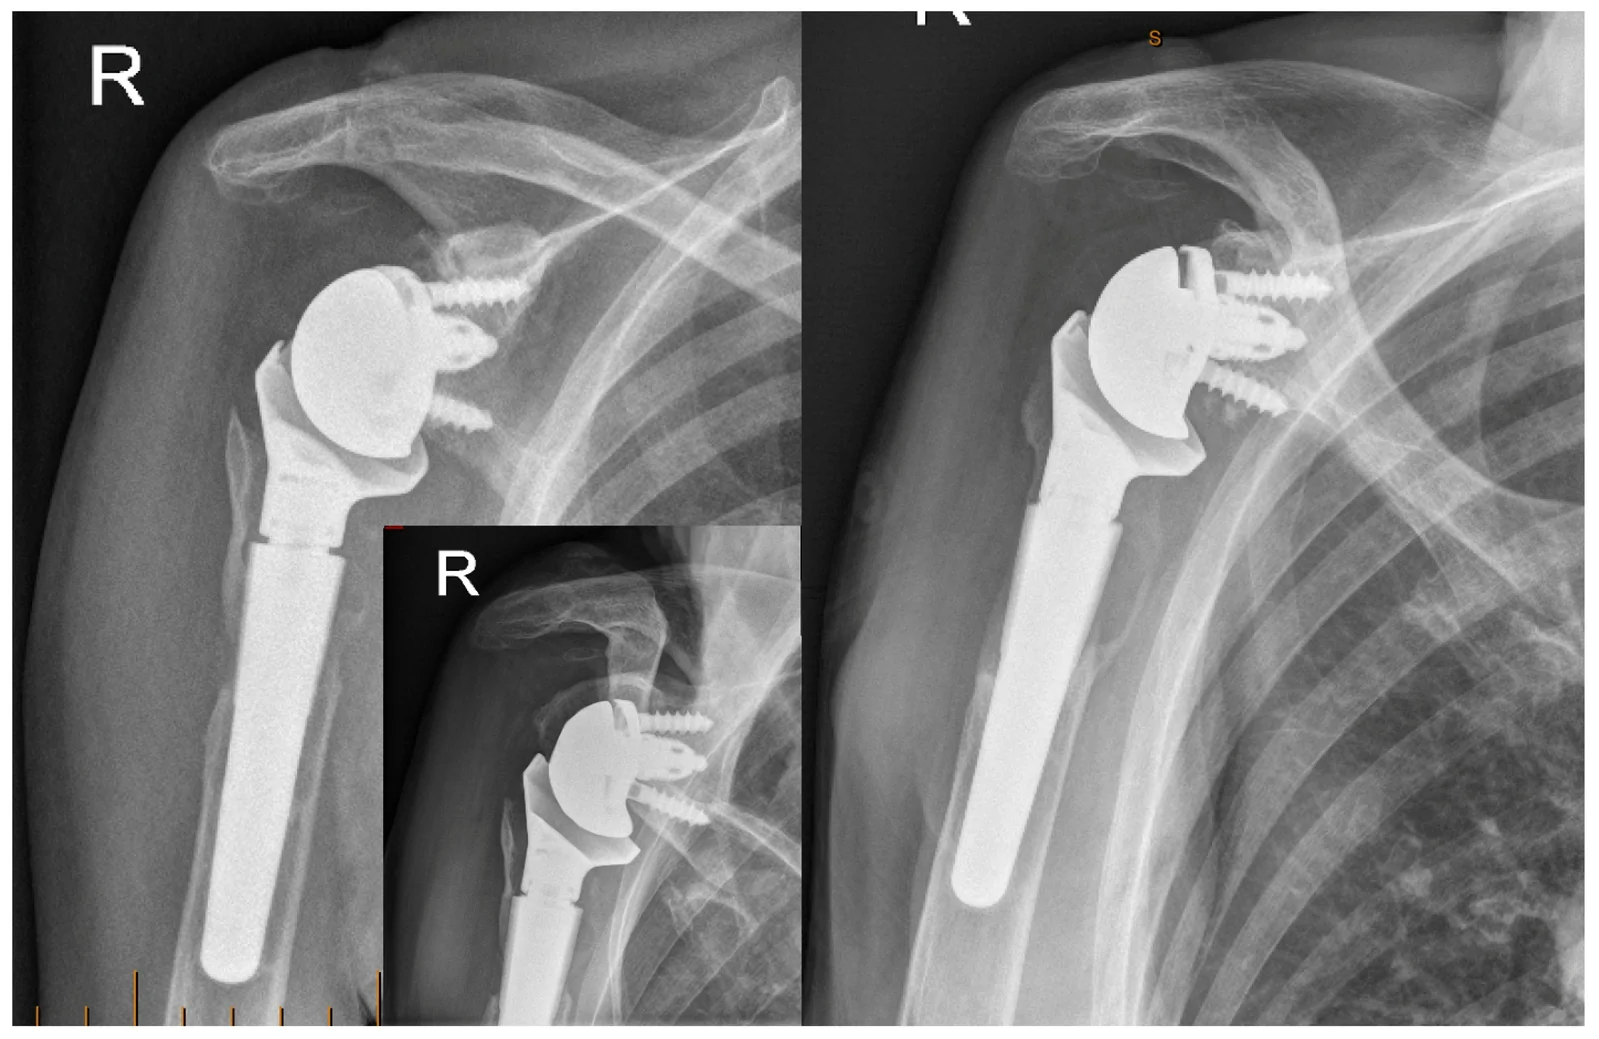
Radiograph of patient with a metal glenosphere taken at 10 and 16 years postoperatively. Note notching progressing to grade 3, with progression of stress shielding around humeral component.
